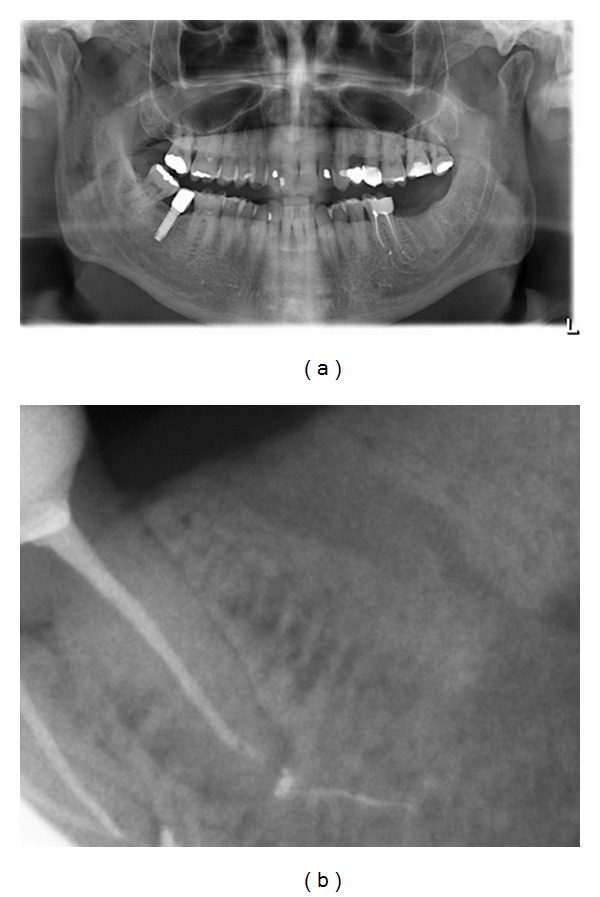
(a) Panoramic radiograph. Notice the correct healing of the socket after 6 months of the molar 37 extraction. (b) Periapical radiograph. Observe the satisfactory bone density in the socket after 6 months of extraction.
